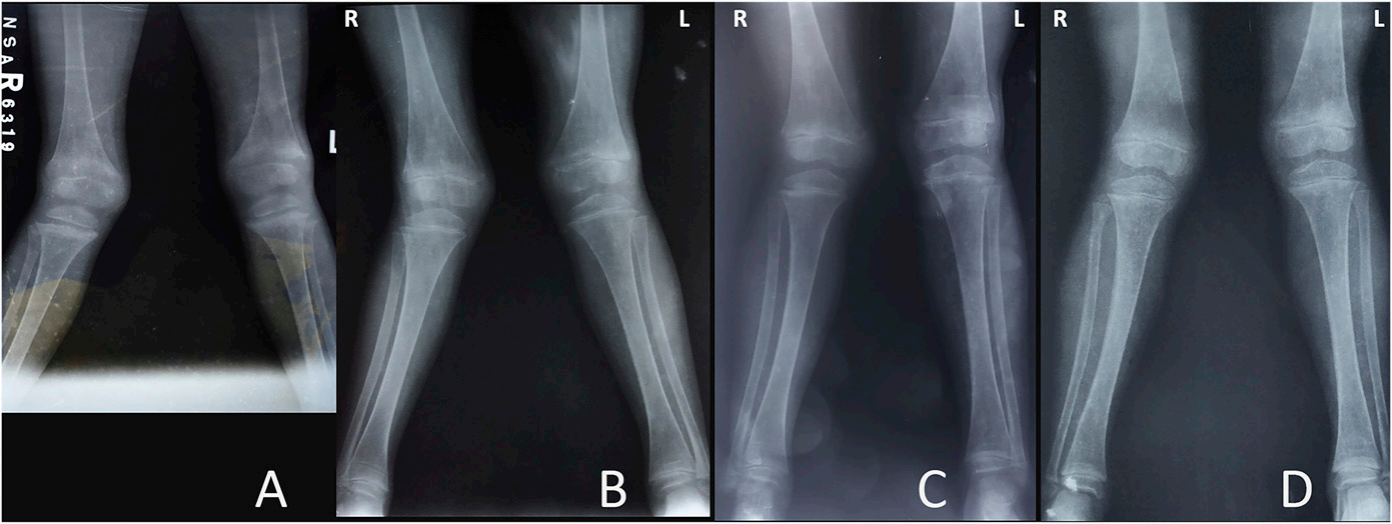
X-rays of a 6-year-old girl. (A) Pre-treatment X-ray shows severe widening/cupping of the metaphyseal growth plates with sclerosis, hypertrophic growth plates, bowing of the diaphyses, and diffuse osteopenia. (B–D) Post-treatment X-rays show a gradual straightening of the diaphysis, reduction in sclerosis at the epiphyses, and normalization of the distance between the distal metaphyses and epiphyses over time.
